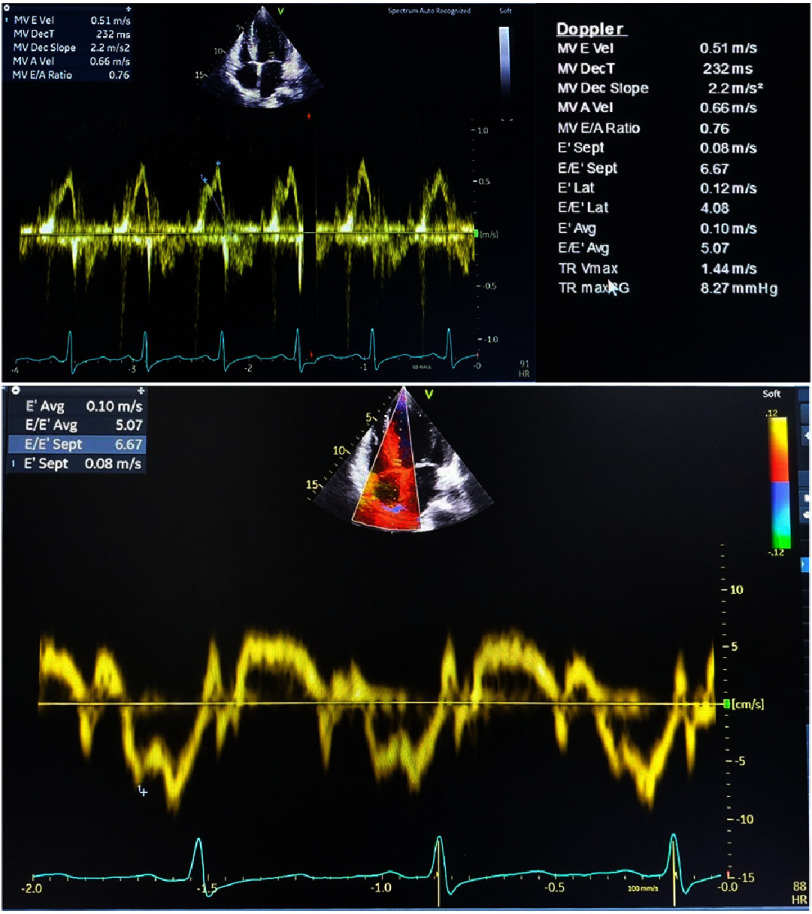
Doppler echocardiography. The E-wave represents early diastolic passive filling of the left ventricle, while the A-wave represents late diastolic blood flow generated by atrial contraction. In this patient, the TR Vmax was 1.44 m/s.
